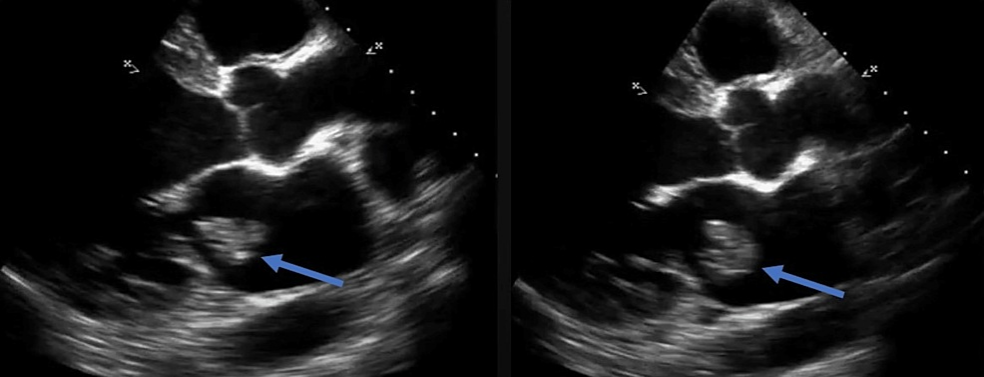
Transthoracic echocardiography demonstrating intracardiac mass in the left atrium, suggestive of atrial myxoma (blue arrows)
